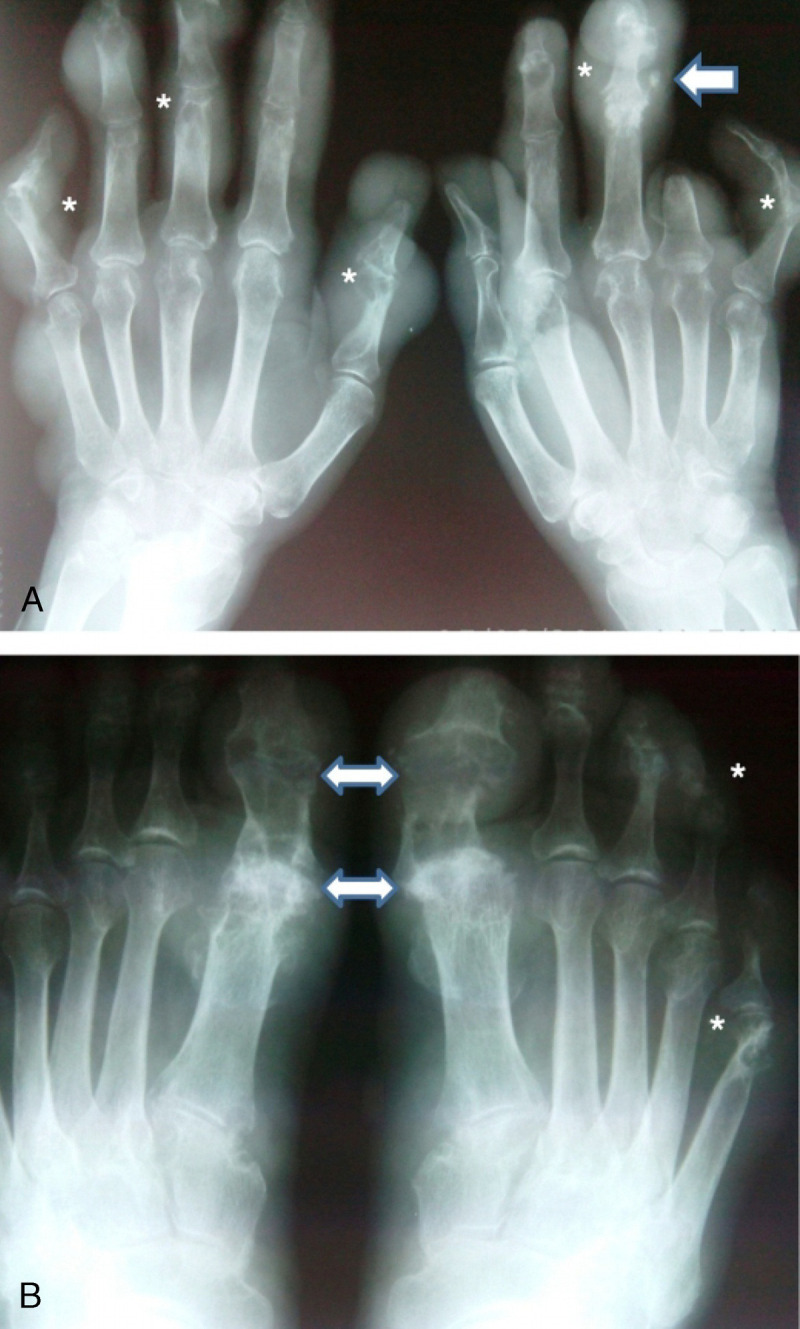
A, Anteroposterior radiograph of the hands: calcified tophus (arrow), suggesting renal impairment. Multiple bilateral interphalangeal articular erosions, adjacent to tophus (asterisk). B, Anteroposterior radiograph of the feet: multiple bilateral interphalangeal (asterisk) and metatarsophalangeal joints erosions (left-right arrow).
